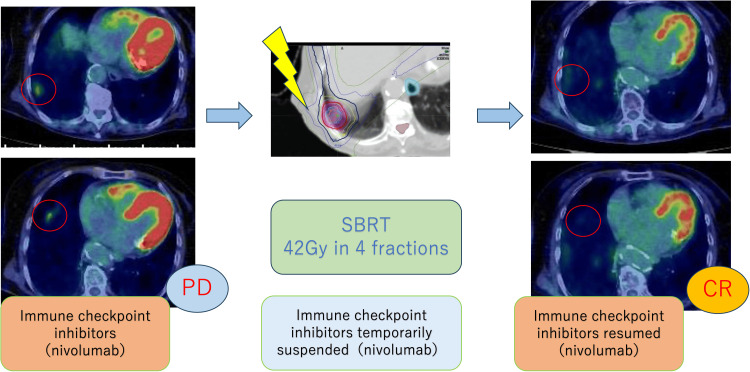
Second abscopal effect observed after SBRT to right lung S9 lesion PET-CT images of the second abscopal effect. Four months after SBRT was performed on the right lung S9 lesion, the metastatic lesion in the right lung S4 disappeared on PET-CT, indicating an abscopal effect SBRT: stereotactic body radiotherapy; PET-CT: positron emission tomography-computed tomography
